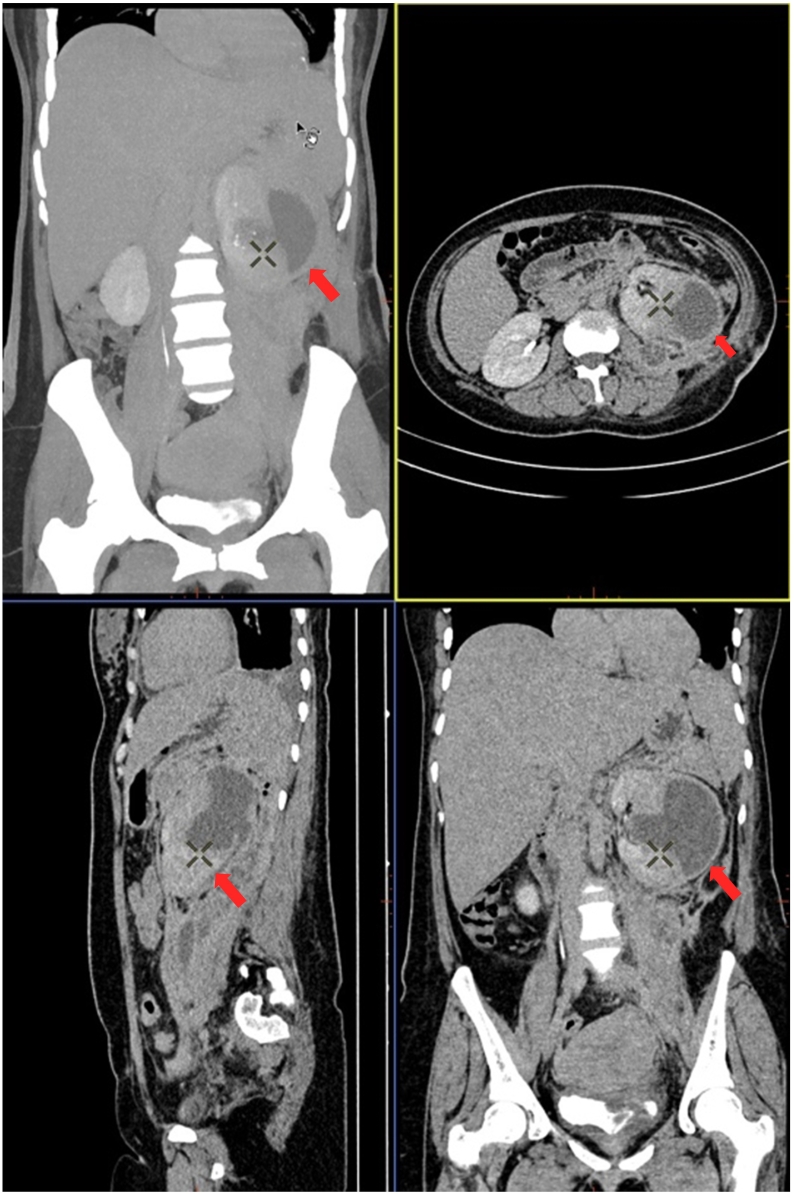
CT SCAN abdomen with contrast showed (red arrow) hyperdense lesion, size ± 7.4 × 7.0 × 7.0 cm, appears to compress the left renal pelvis. (For interpretation of the references to colour in this figure legend, the reader is referred to the web version of this article.)
